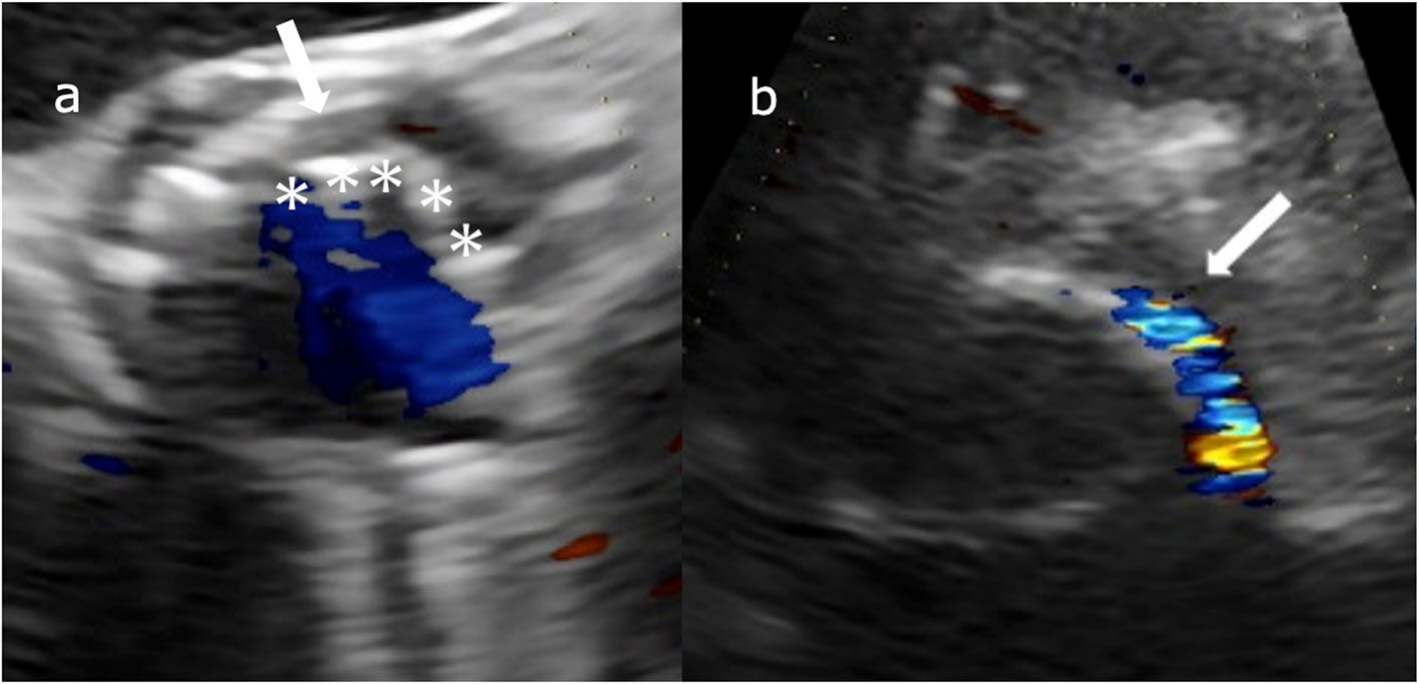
a Postoperative transesophageal echocardiography showing a gap between the TAVI valve (asterisk) and the native annulus (arrow), indicating incomplete apposition of the valve. b Color Doppler imaging demonstrating mild paravalvular leak (PVL) (arrow)
